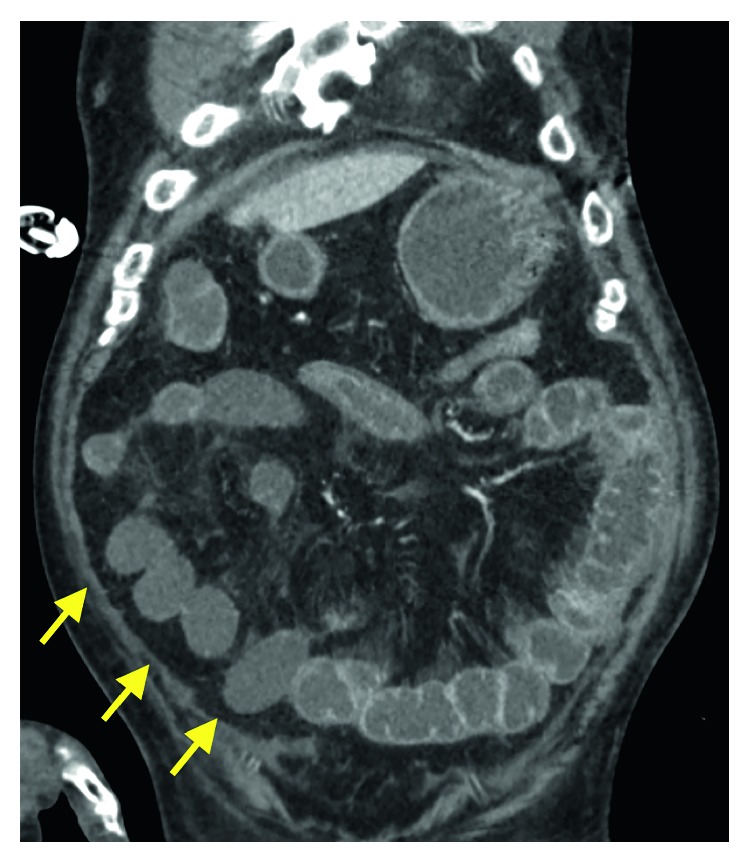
Coronal-section contrast-enhanced CT of the abdomen performed in the ICU. Poor contrast effects can be seen in the small intestine (arrows).
